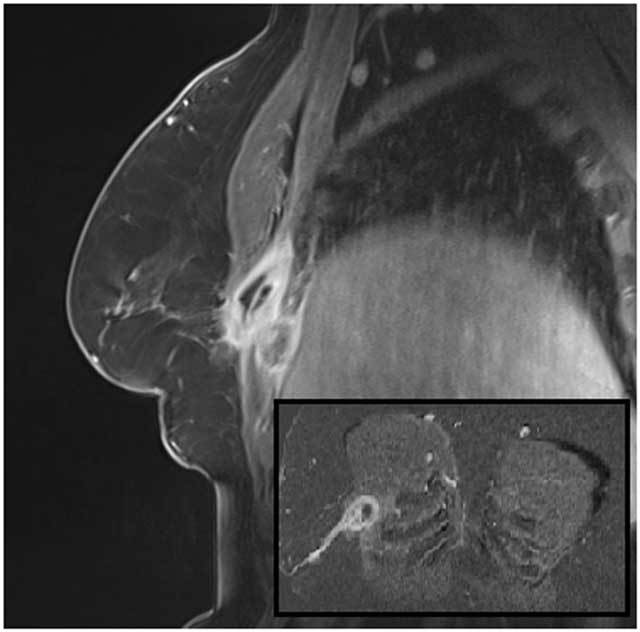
MRI sagittal reconstruction with IV gadolinium contrast. Subfigure: coronal MIP reconstruction.
